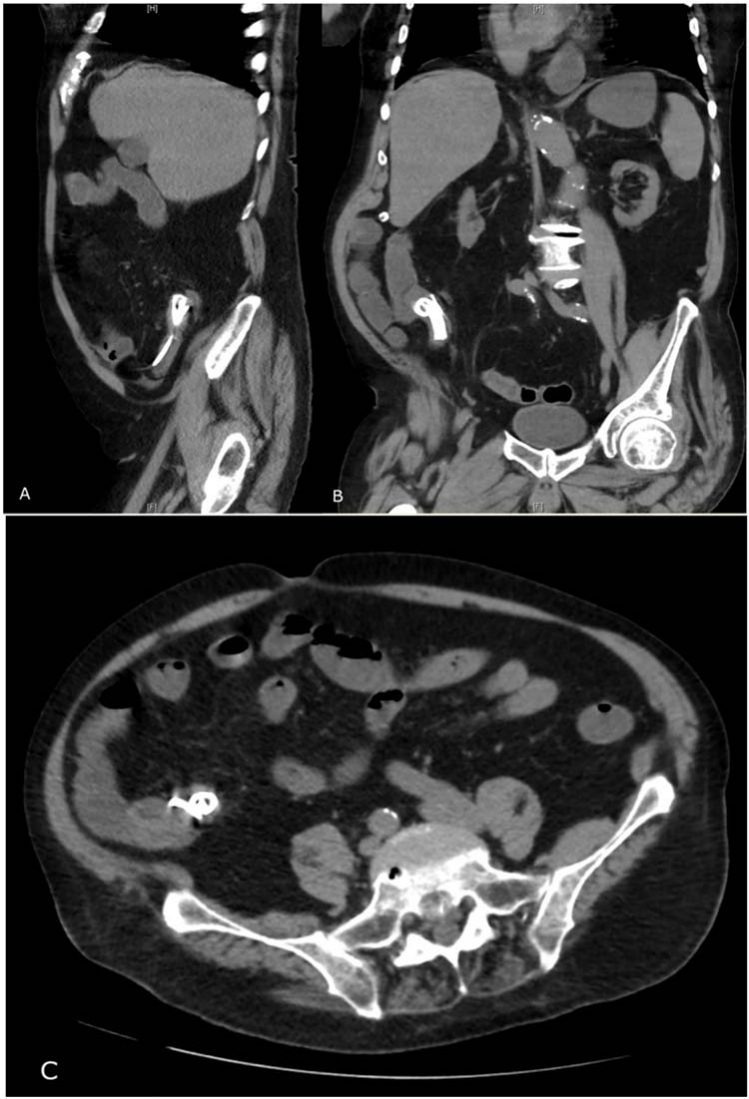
Abdominal CT showing migrated biliary stent in sagittal (A), coronal (B) and transverse (C) views.
